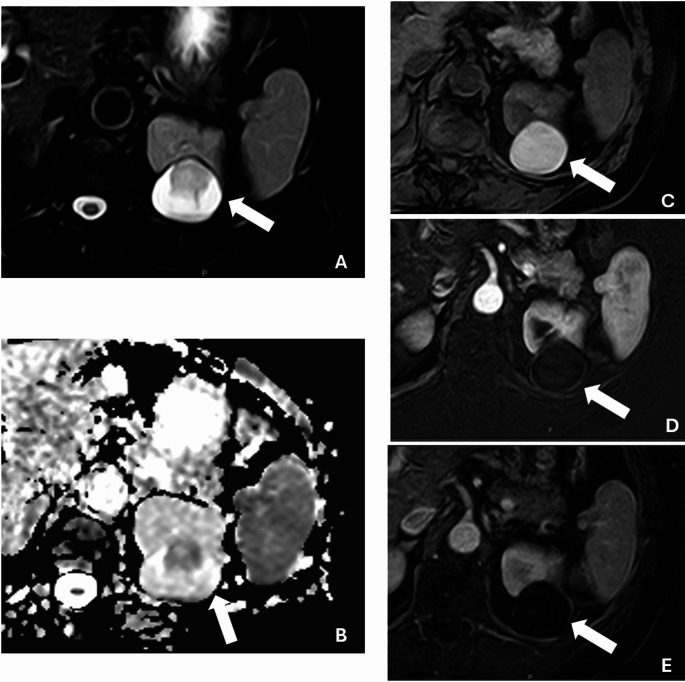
Complex renal cyst in a 68-year-old female with HLRCC. Contrast-enhanced MRI reveals a well-circumscribed complex cyst measuring 4.0 cm (arrows), demonstrating heterogeneous T2 intensity on the T2-weighted image (A). The cyst exhibits restricted diffusion on the ADC map (B) and appears hyperintense on the pre-contrast phase (C). No enhancement is observed on the subtracted images of the post-contrast nephrogenic (D) and delayed (E) phases. Final pathology confirmed the cyst as benign.
